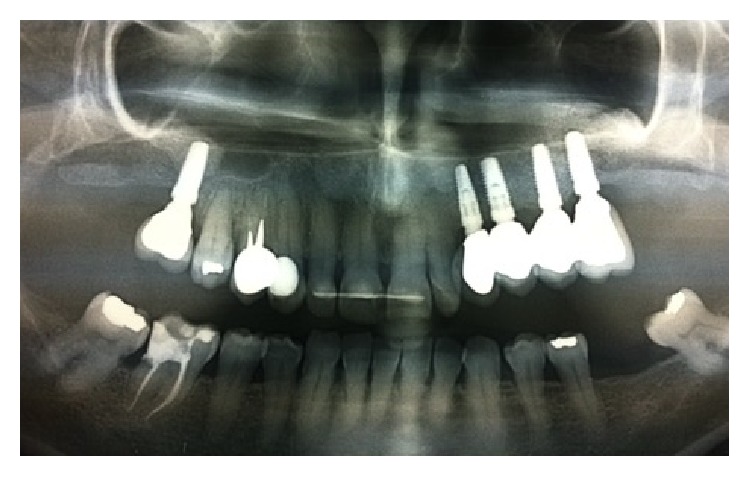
Two-year postoperative X-ray control evidences the integration of the dental implants for support of a fixed prosthetic rehabilitation.
